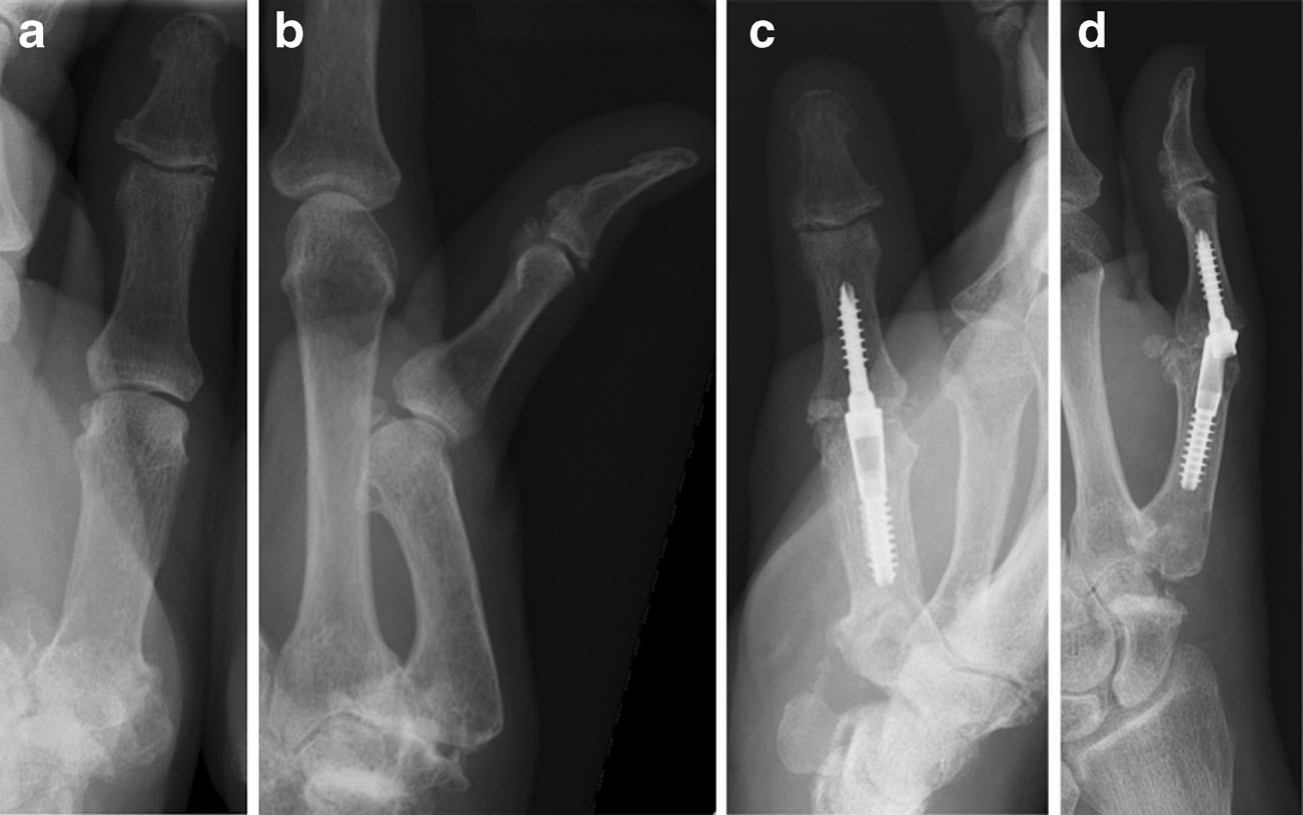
a Preoperative AP radiograph, left thumb of 60 yo female with trapeziometacarpal osteoarthritis and metacarpophalangeal joint instability (Pt. 6). b Preoperative lateral radiograph, left thumb of Pt. 6. c Postoperative (4 weeks) AP radiograph, left thumb of Pt. 6 showing intramedullary compression device and fusion of metacarpophalangeal joint. Postoperative (4 weeks) lateral radiograph, left thumb of Pt. 6 showing intramedullary compression device and fusion of metacarpophalangeal joint
